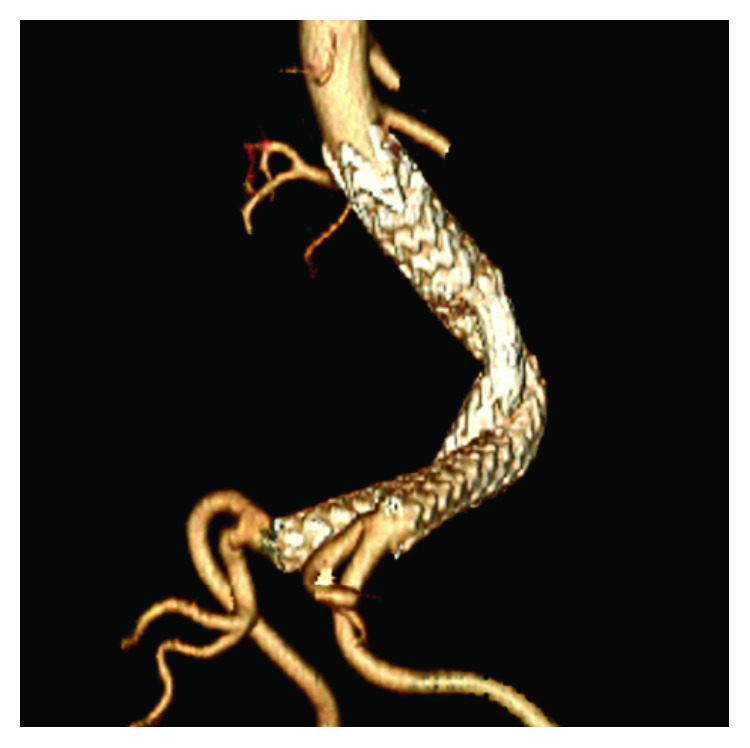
Surveillance follow-up CTA at 18 months. Three-dimensional reconstruction demonstrates the correct placement of the bilateral iliac limbs at the seal zones without evidence of endoleaks.
